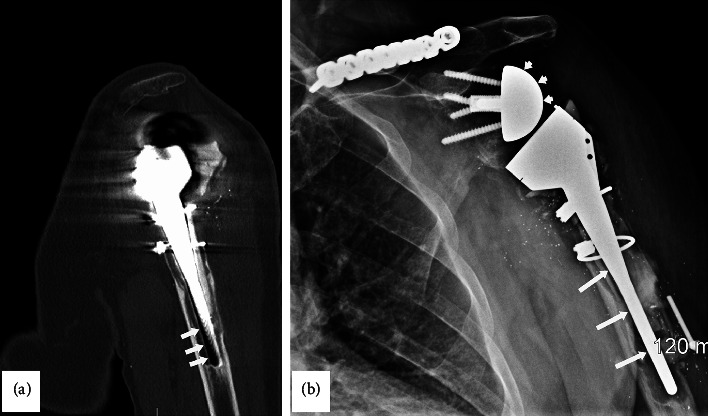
(a) CT scan of the left shoulder that shows the metal prosthesis of the upper half of the humerus. Note erosion of the bone surrounding the lower end of the prosthesis (arrows). (b) AP radiograph of the left shoulder demonstrating the arm and shoulder with bone loss surrounding the lower end of the prosthesis (arrows).
